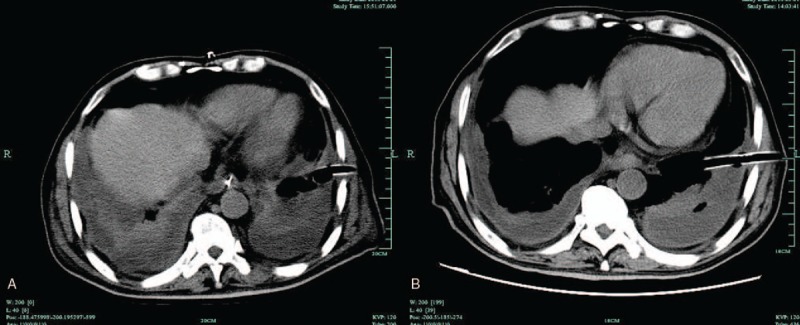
(A) Thoracic computed tomography (CT)-scan: multiperforated pleural drainage tube was positioned for patients with pleural effusion or empyema. (B) Thoracic CT-scan: effusion or empyema reduced observably after closed thoracic drainage.
